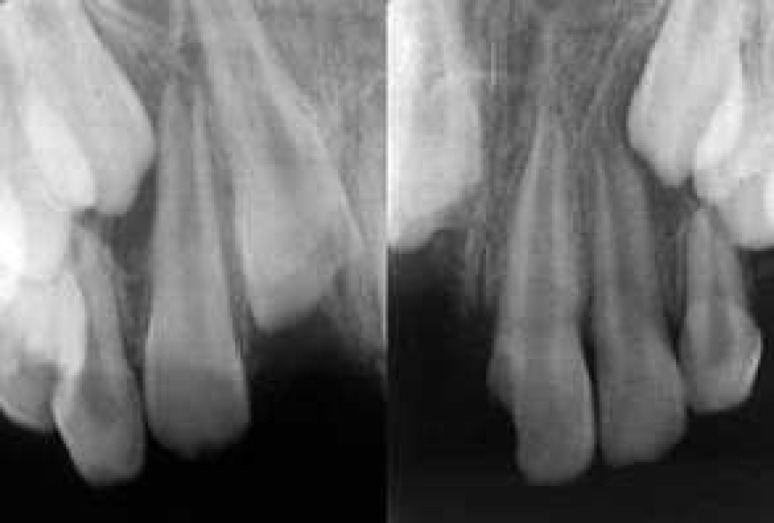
Periapical radiographies shows complete intrusion of right maxillary incisor
